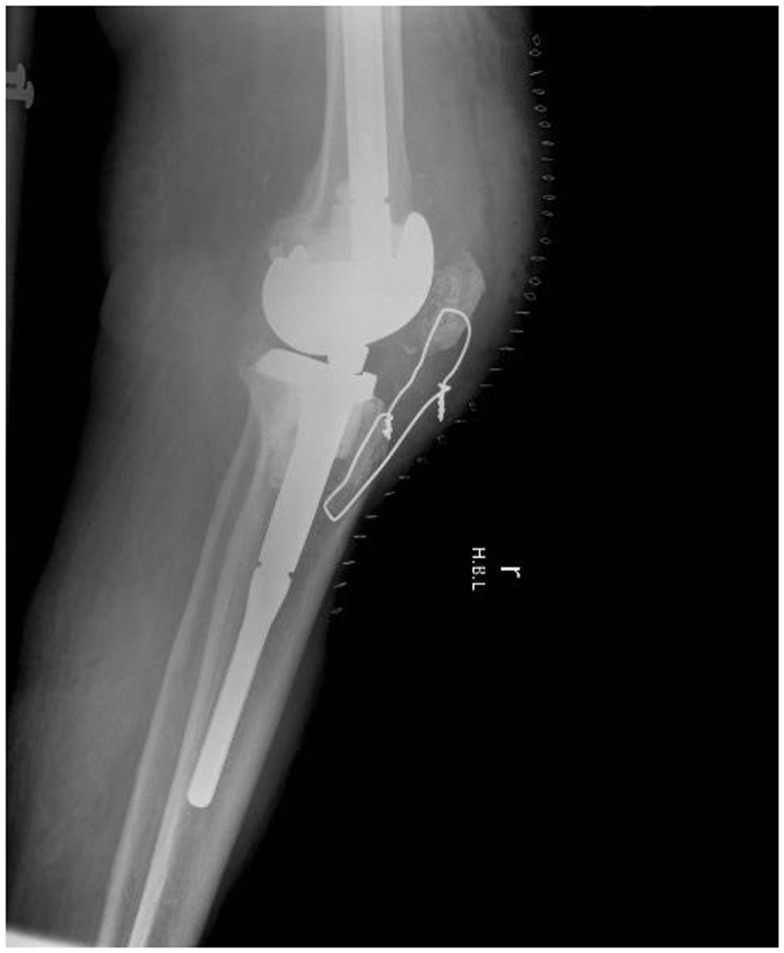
Lateral radiograph showing a hinged knee prosthesis and a cerclage wire augmenting the patella tendon repair in Case 2.
